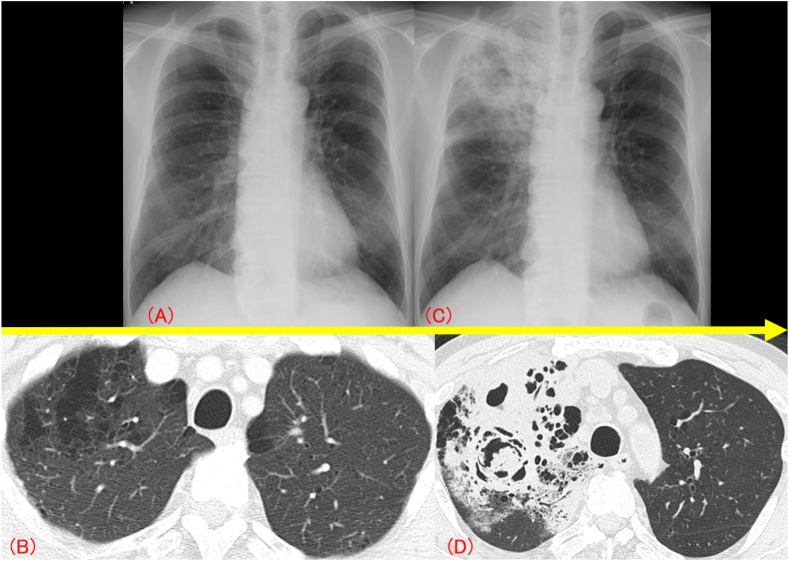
Chest radiographs (X-rays) and computed tomography (CT). On the patient's first presentation, his chest X-ray (A) and chest CT (B) revealed a diffuse emphysematous lesion without any infiltration. At the second visit, his X-ray (C) showed consolidation with a cavity in the right upper lung field, and chest CT (D) showed consolidation with an air-crescent sign.
